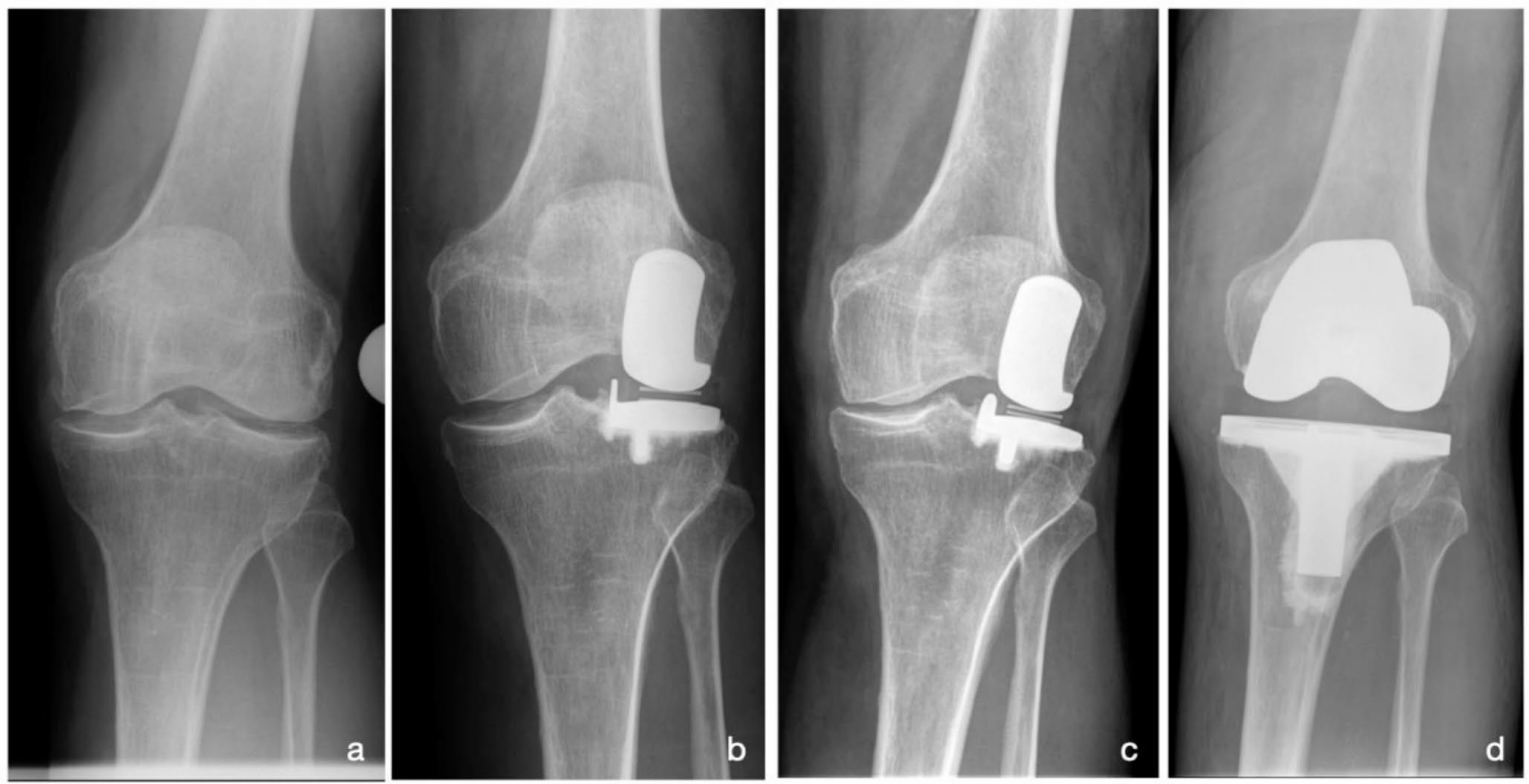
Case history of a 75-year-old male patient who developed progressive medial OA following the implantation of a lateral UKR (c), which ultimately required conversion to TKR 27 months after the initial surgery (d). As shown in radiograph b, the tibial bone resection appears to be very small, possibly causing an overcorrection of the knee alignment that may have contributed to the early progression of medial OA. Although the presented radiographs suggest preexisting OA in the medial compartment, the intraoperative assessment during the index surgery confirmed the indication for lateral UKR. a: Weight-bearing AP x-ray of the left knee prior to the initial surgery. b: Postoperative AP x-ray shortly after UKR. c: AP x-ray 27 months after lateral UKR, showing significant medial OA progression. d: AP x-ray after revision surgery to TKR. AP: antero-posterior, OA: osteoarthritis, TKR: total knee replacement, UKR: unicompartmental knee replacement
